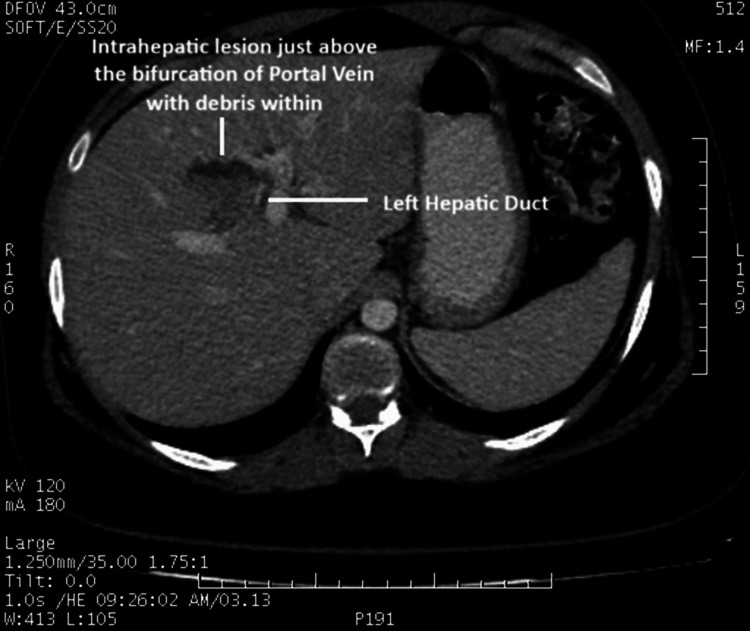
Axial CT scan showing the intrahepatic lesion with debris.
